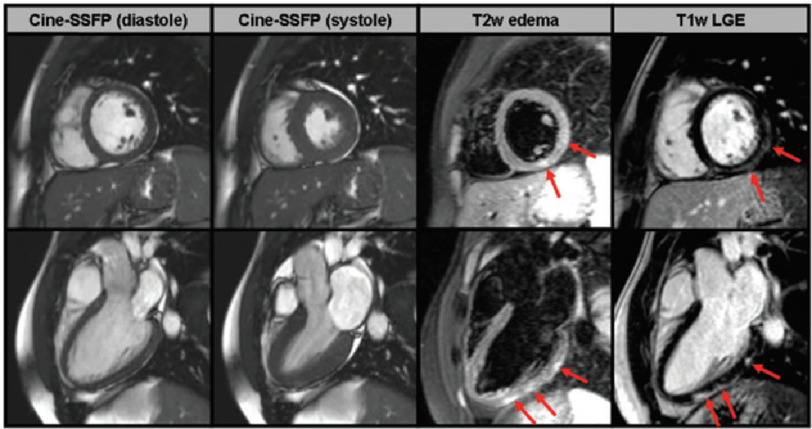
Short-axis (upper line) and long-axis (lower line) CMR images of a young patient with acute myocarditis. In the first two columns, cine-SSFP images are shown in diastole and systole and suggest absence of any wall motion abnormality. In the next column, T2-weighted edema images demonstrate the presence of patchy focal edema in the sub-epicardium of the inferolateral wall (red arrows). In the last column, T1-weighted LGE images demonstrate presence of sub-epicardially distributed LGE (red arrows) which is typical for acute myocarditis^19^.
